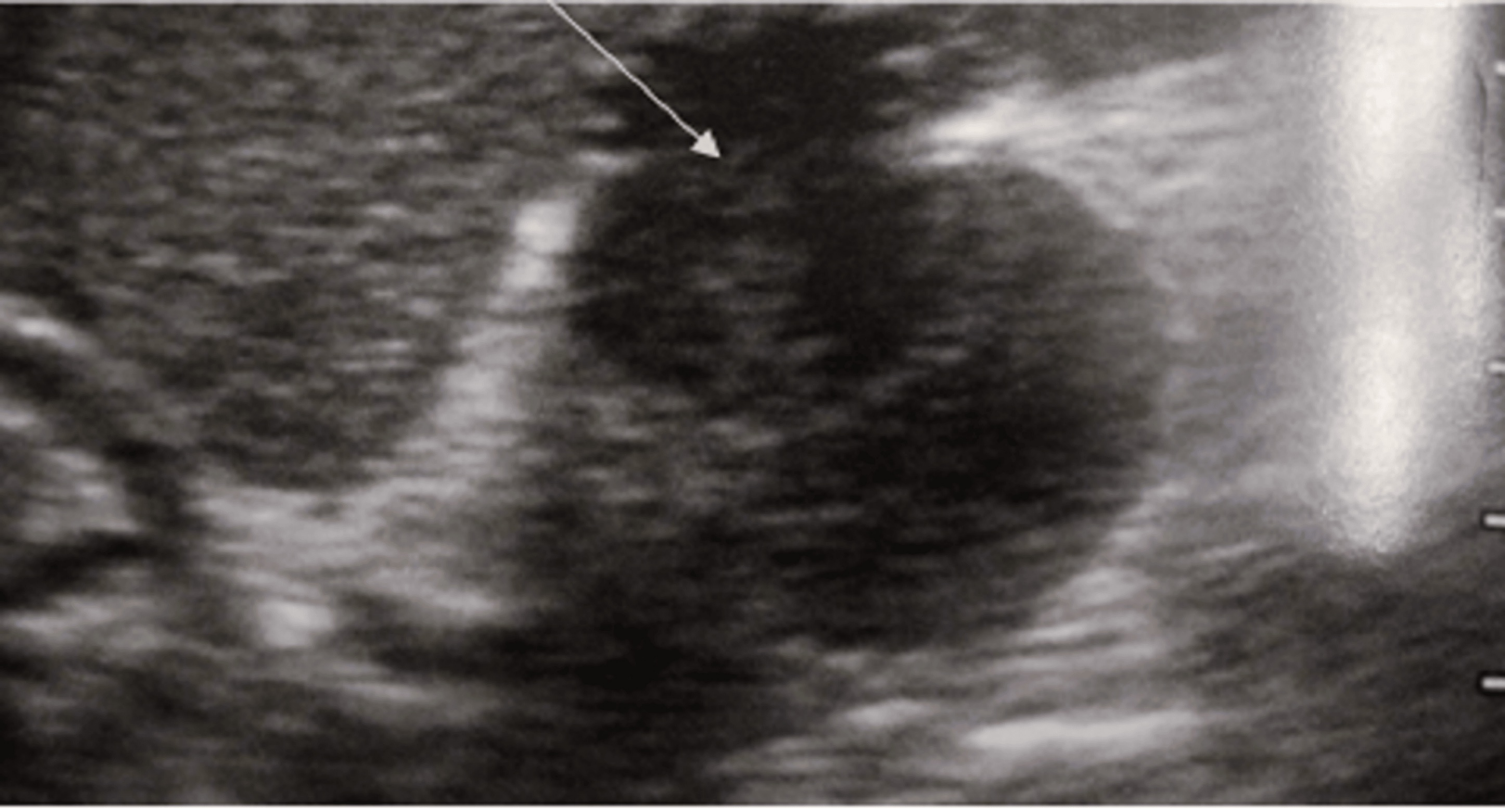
Ultrasound image of the patient showing acute cholecystitis with small gallstones.
